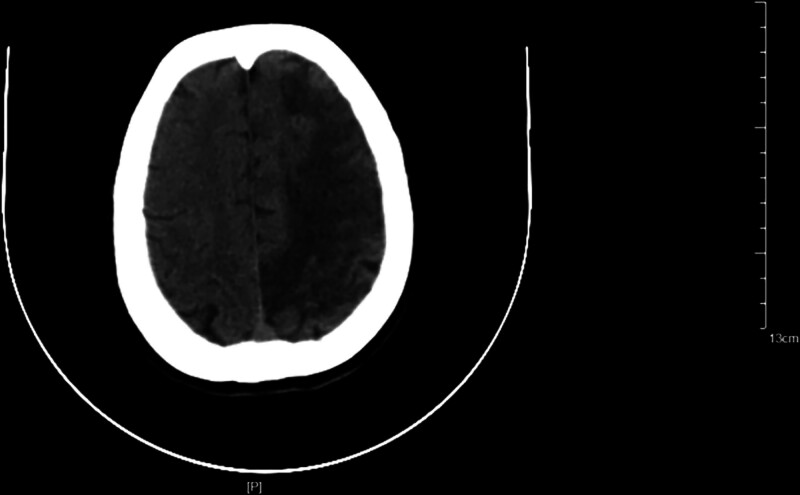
Brain computed tomography (CT) scan from Patient 2 at admission, showing a left frontal-parietal infarction and multiple bilateral lacunar infarctions.
